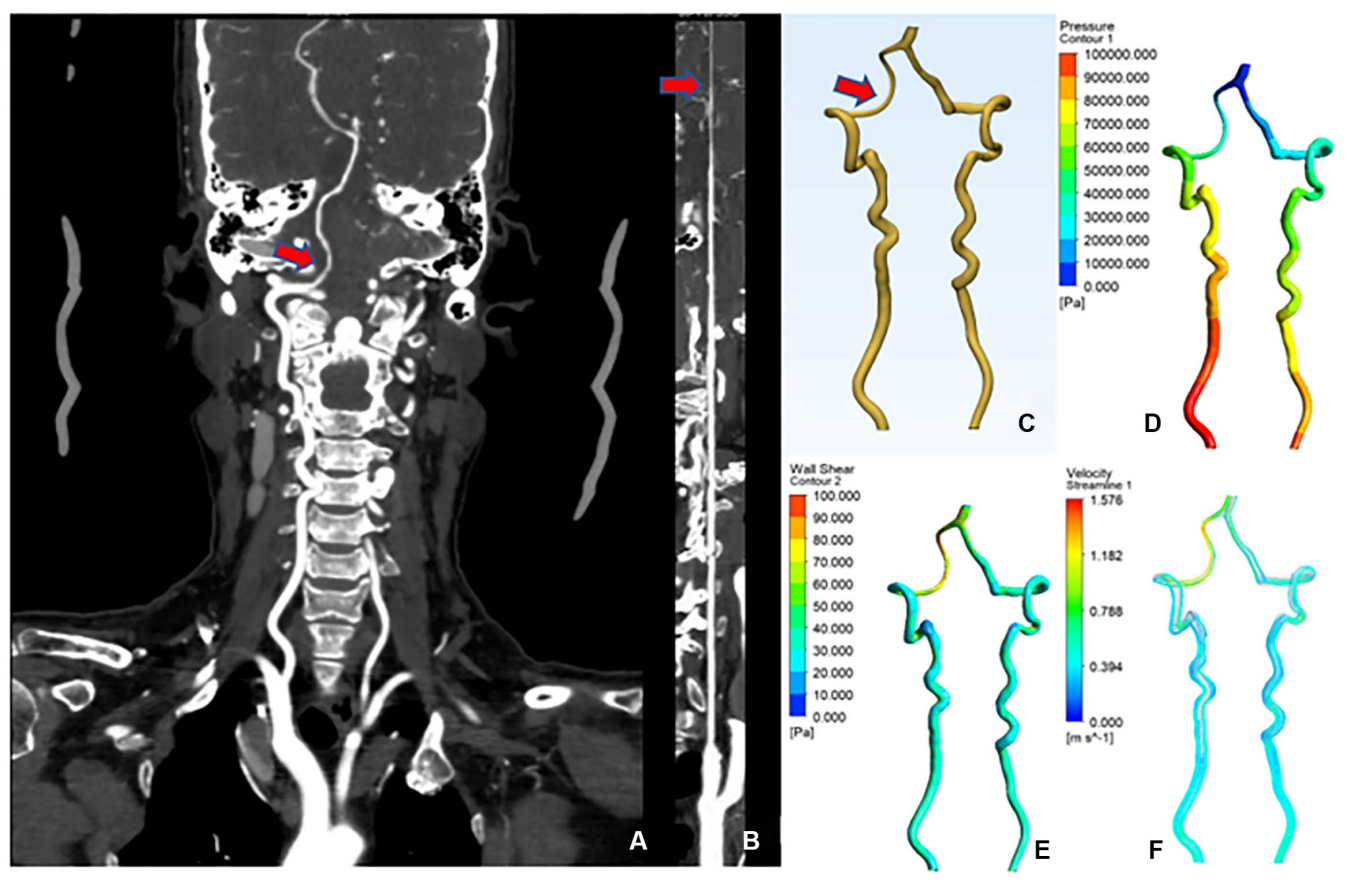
CTA and CFD images of vertebral artery in case 1. (A) Vertebral artery CTA coronal position. (B) Straightened right vertebral artery. (C) CAD model of vertebral artery after smoothing process. (D) Changes in wall pressure gradient at the vertebral artery. (E) Changes in wall shear gradient at the vertebral artery. (F) Vertebral artery flow velocity streamline.
